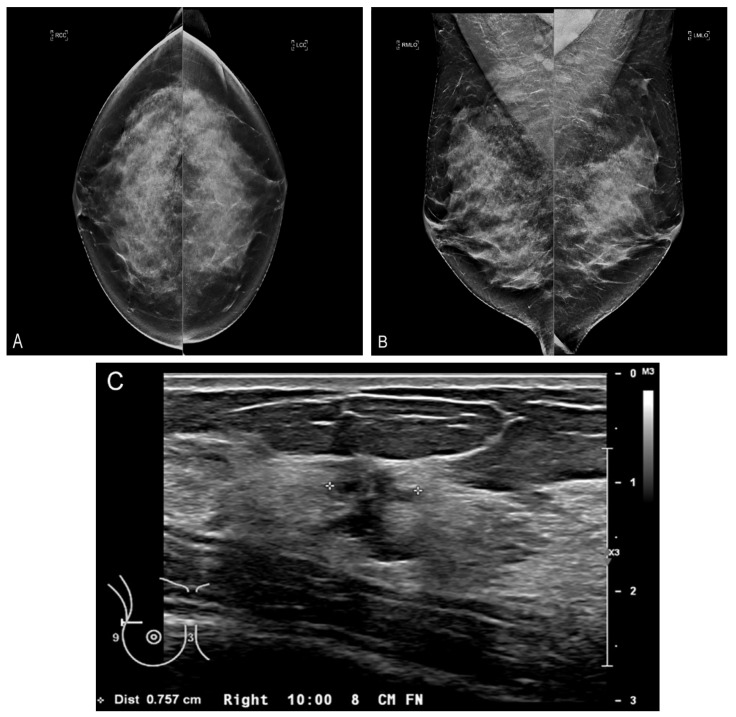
Limitation of mammography for dense breasts. Bilateral craniocaudal (CC) (A) and bilateral mediolateral oblique (MLO) (B) mammograms showing extremely dense breasts (ACR density category d) [7]. The mammogram is negative (Breast Imaging Reporting and Data System (BI-RADS) 1). (C) Fortunately, the patient had a screening ultrasound shortly thereafter showing a 1 cm cancer at the 10 o’clock position in the right breast. The mammogram was a false negative. The patient came to no harm since the ultrasound was performed within a short period of time. The cancer was grade I invasive ductal carcinoma with negative nodes. Survival after treatment is near 100% [8].
